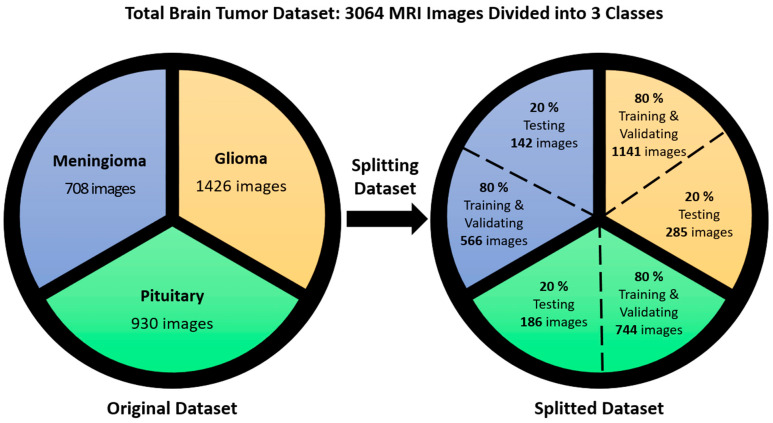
Distribution of meningioma, glioma, and pituitary MRI images in training, validation, and testing dataset.
